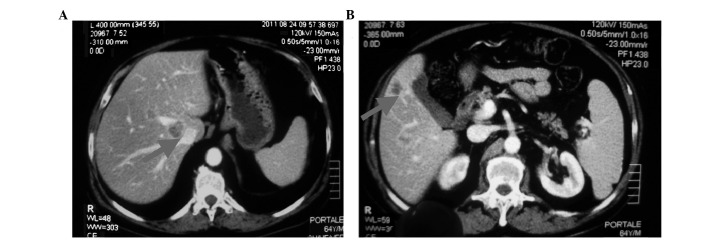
Computerised tomography (CT) scan performed on August 24th 2011, demonstrating liver metastases with maximum diameters of 3 cm (A) and 2 cm (B).
